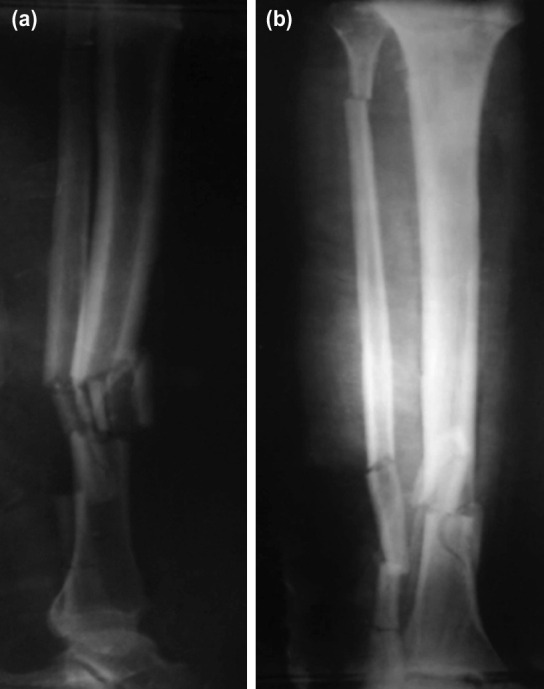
(a) Lateral and (b) anterior posterior plain radiograph of open fracture of the tibia.
